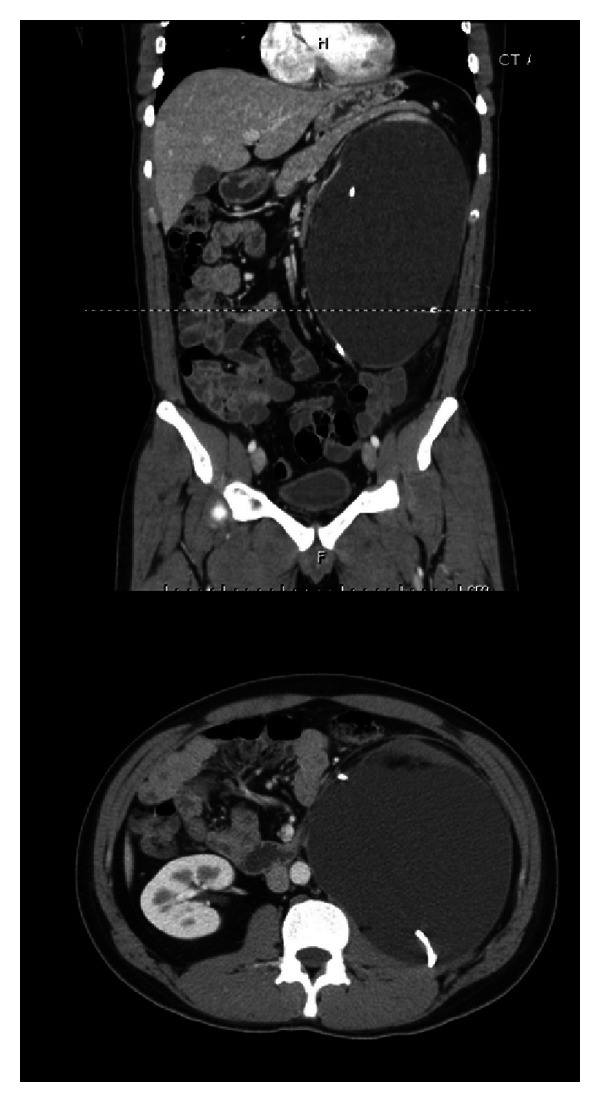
Persistent urinoma seen on repeat CT abdomen and pelvis with contrast after placement of percutaneous nephrostomy tube and ureteral stent.
